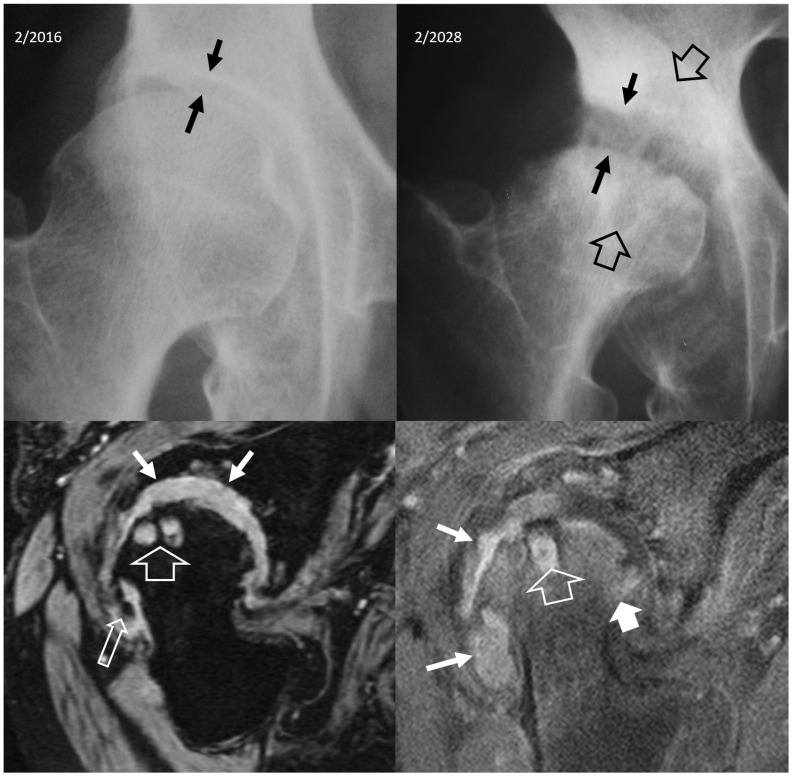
Rapidly destructive osteoarthritis of the hip in a 72-year-old female patient with constant pain and limited range of motion. A plain radiograph (upper left) taken with the onset of symptoms shows a normal joint space (arrows). Two years later, a plain radiograph shows the widening of the joint space (arrows) and subarticular cyst formation (open arrows). Oblique axial gradient echo (lower left) and fat-suppressed contrast-enhanced T1-w (lower right) MR images showing subarticular cysts (open arrows), osteophyte formation (thin open arrow), synovitis (arrows), and bone marrow edema (thick arrow).
